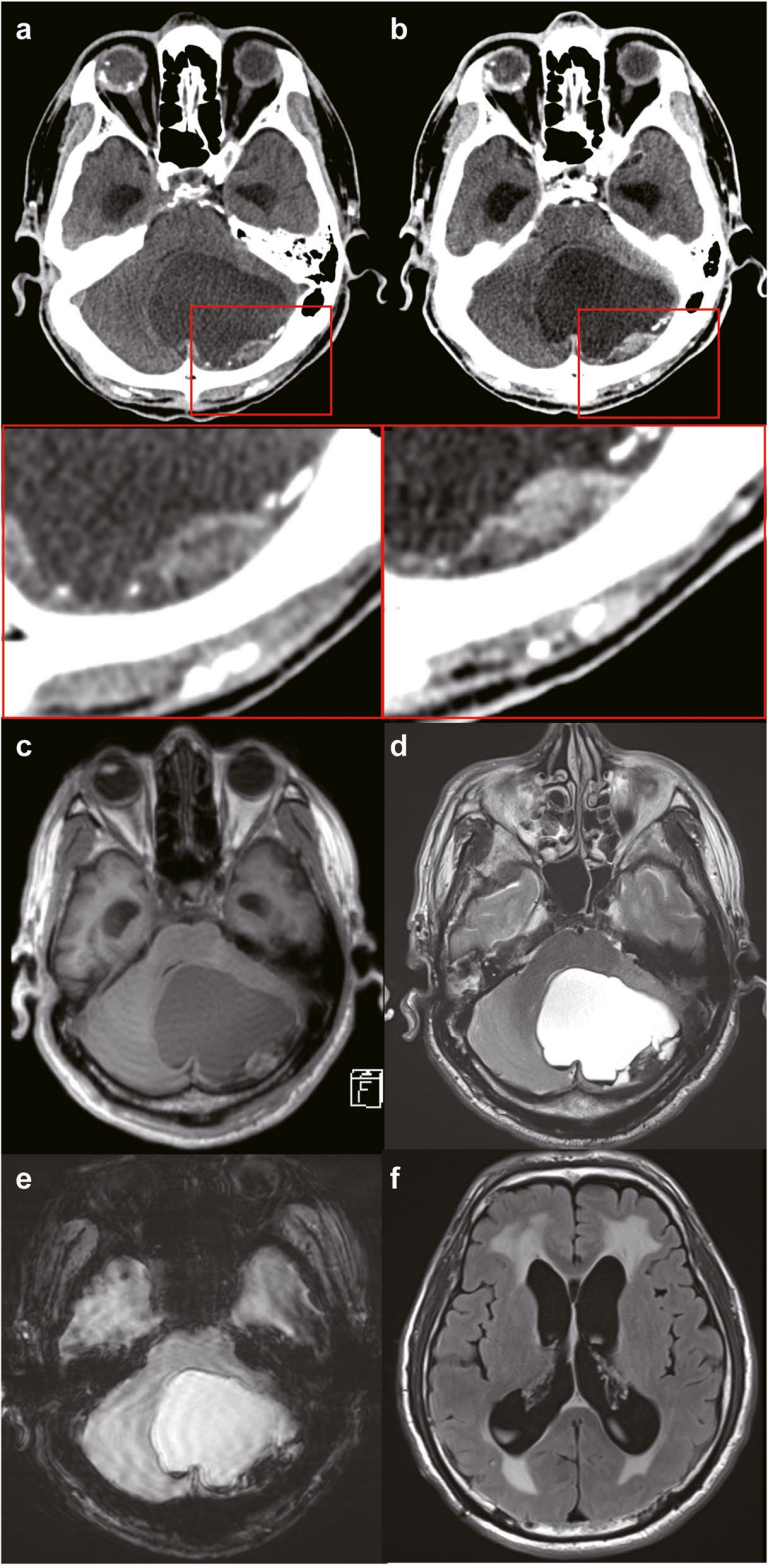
Pre-operative images of computed tomography (CT) scans and magnetic resonance imaging (MRI). a CT revealed a cystic lesion with a mural nodule in the left hemisphere of the cerebellum. The lower panel shows a magnified image of the area within the red rectangle in the upper panel. The nodular component was accompanied by a high attenuated area, suggesting focal calcification. Hydrocephalus was associated with a shift of the fourth ventricle to the right. b Contrast-enhanced CT showed mild enhancement in the mural nodule. The lower panel shows a magnified image of the area within the red rectangle in the upper panel. c, d MRI demonstrated that the cystic lesion was hypointense on a T1-weighted image (c) and hyperintense on a T2-weighted image (d). The tumor was well-defined, and no peritumoral brain edema was present. e T2 star-weighted image shows a hemorrhage within the cyst and in the mural nodule. f Fluid-attenuated inversion recovery image shows hydrocephalus with periventricular hyperintensity
